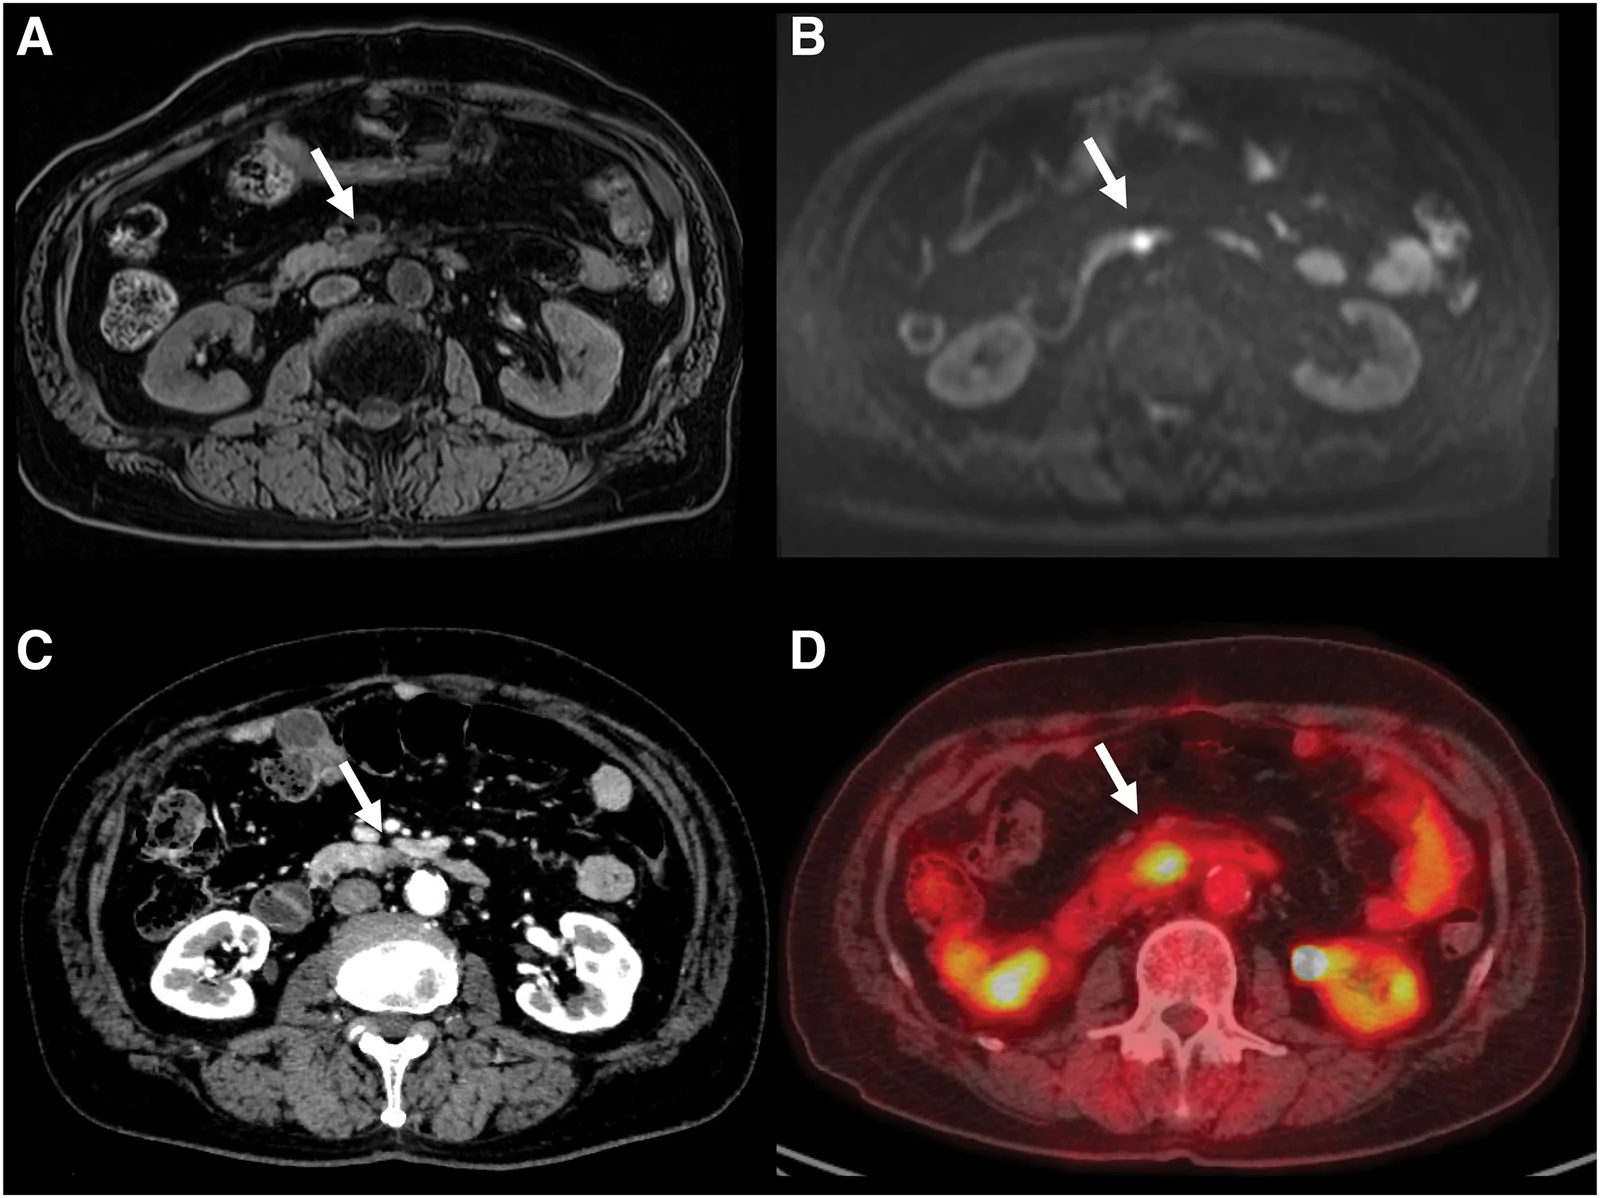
Uncinate process of the pancreas on MRI, CECT, and ^18^F-FDG PET-CT. (A) T1-weighted image showing a 2.7-cm hyperintense mass (arrow). (B) Diffusion-weighted image showing a hyperintense mass (arrow). (C) CECT showing a poorly circumscribed, hypovascular lesion (arrow). (D) ^18^F-FDG PET-CT showing FDG uptake (SUVmax 5.0, arrow). ^18^F-FDG, fluorine-18 fluorodeoxyglucose; CECT, contrast-enhanced CT; SUVmax, maximum standardized uptake value
